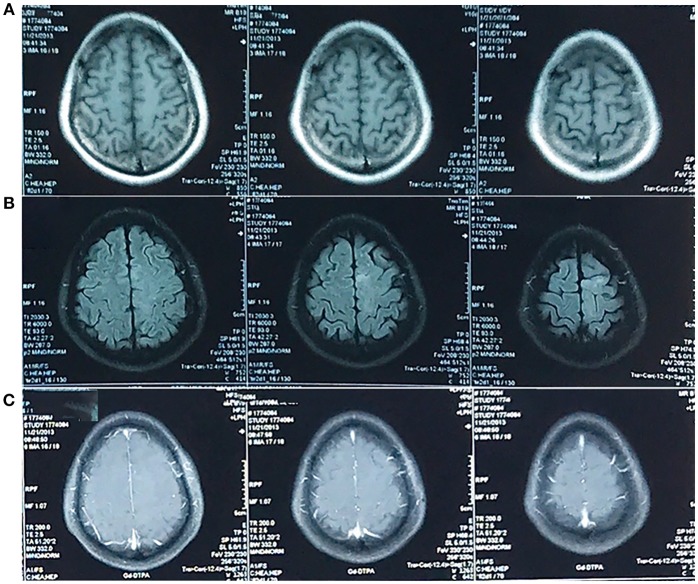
MRI in November 2013. An abnormal signal was confined to left frontal lobe. It was not obvious on the T1-weighted image (A) and hyperintense on the T2-weighted-Fluid-Attenuated Inversion Recovery (T2-FLAIR) image (B) in the absence of enhancement (C). The T2-weighted image was unavailable.
